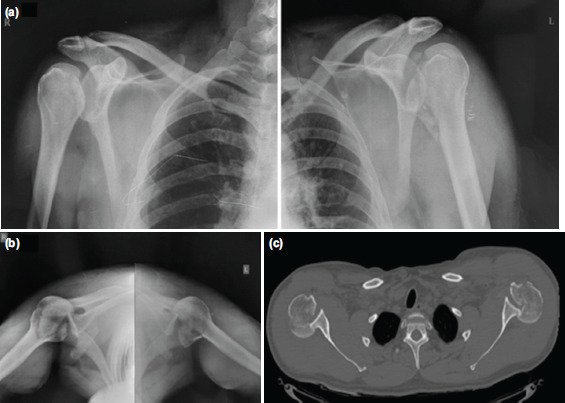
(a) Pre-operative radiographs (a) anteroposterior and (b) axillary and (c) computed tomography of both shoulders showing bilateral fracture-dislocation and defects.
